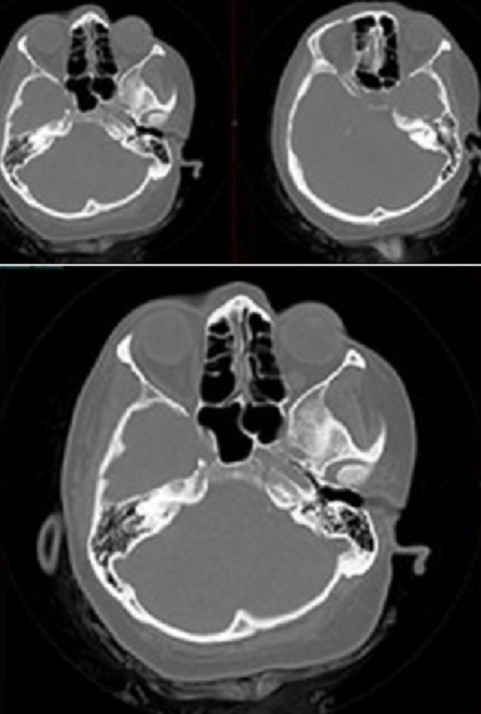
Axial images of paranasal computed tomography that shows no bone defect for rhinorrhea
